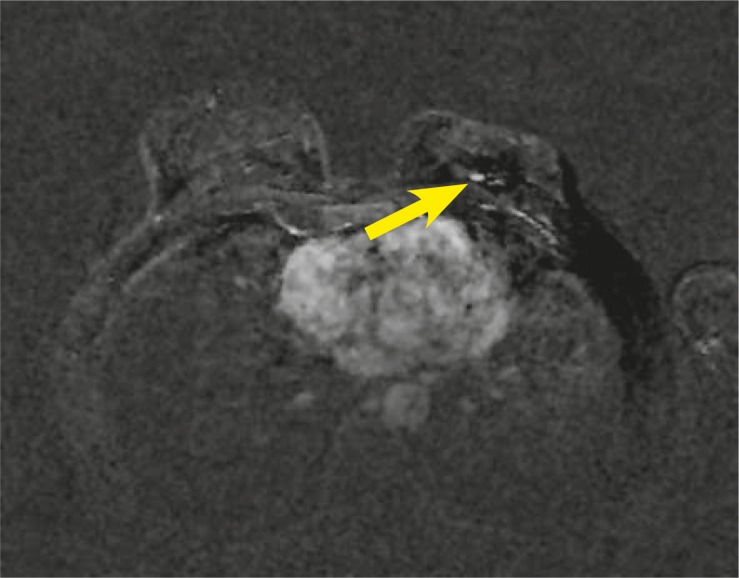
Axial T1-weighted image after Gadolinium injection (2^nd^ minute) and subtraction, focal enhancement 7 × 5 mm in the left breast in the prepectoral region (arrowhead).
